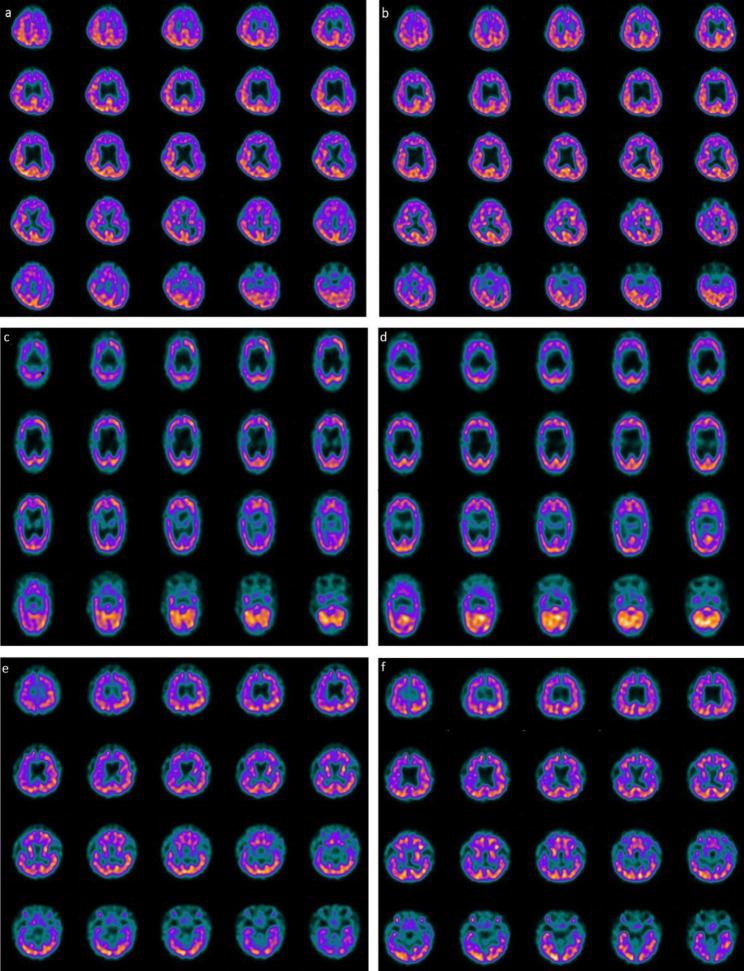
Perfusion SPECT images before and after combined treatment with hr-NGF and tDCS. (a)^99m^Tc-HMPAO SPECT images (transaxial slices) of the first patient, before the combined treatment, showed a moderate reduction in radiotracer uptake (hypoperfusion) in the left frontal and temporal cortices, as well as in the left caudate nucleus, left putamen and right thalamus. (b) After the combined treatment, a mild increase in ^99m^Tc-HMPAO uptake was detected in the left frontal cortex (+ 10%), left temporal cortex (+ 9%), left caudate nucleus (+ 17%), left putamen (+ 13%) and right thalamus (+ 12%). (c)
